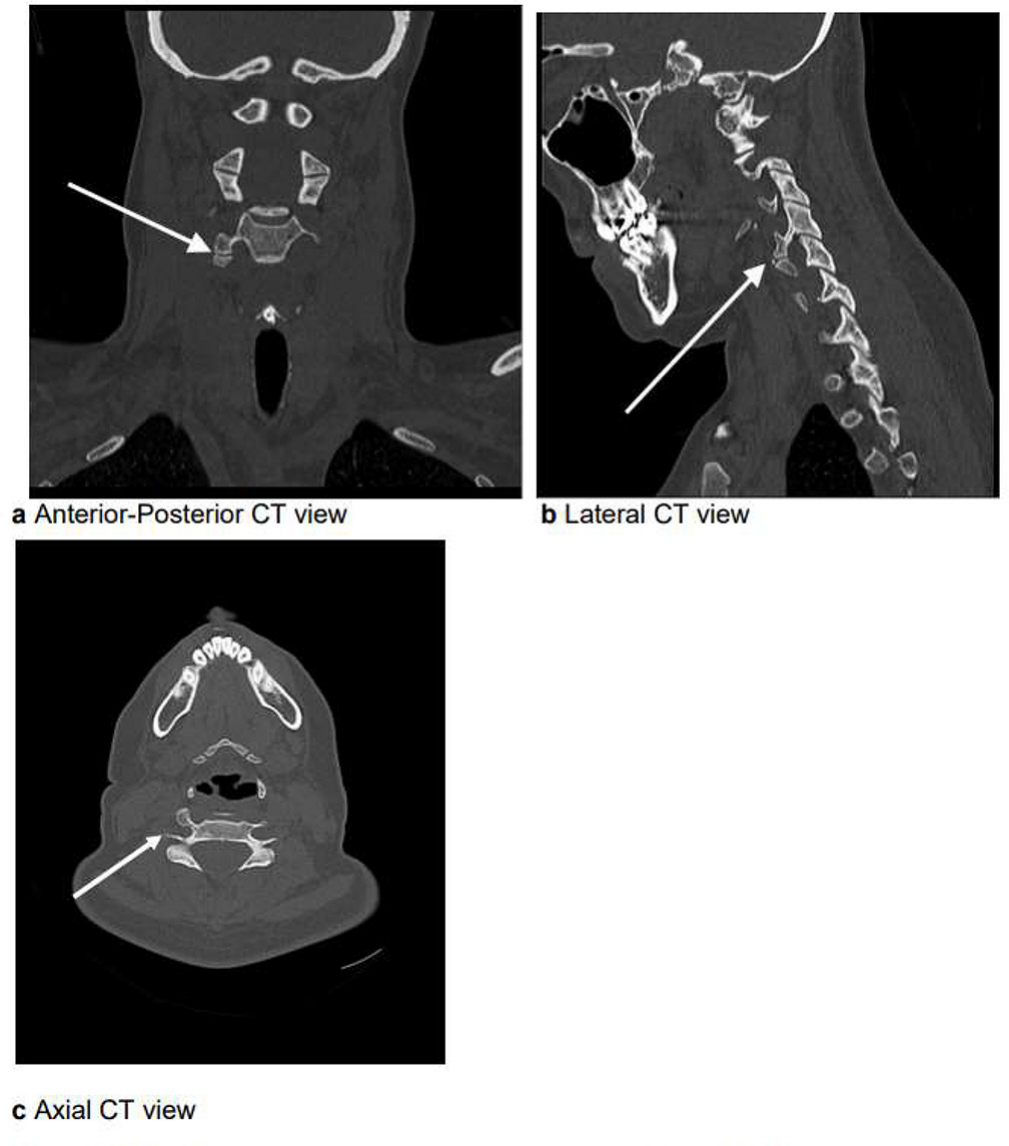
CT scan of C-spine showing an accessory articulation of C4 and C5 right transverse processes, as seen on the lateral cervical spine radiograph. Pathology associated with anomalous transverse processes is uncommon—with particular emphasis on the thoracic vertebrae. There is one case report in English literature with documentation of a T10 thoracic vertebrae with duplicated transverse process.^
